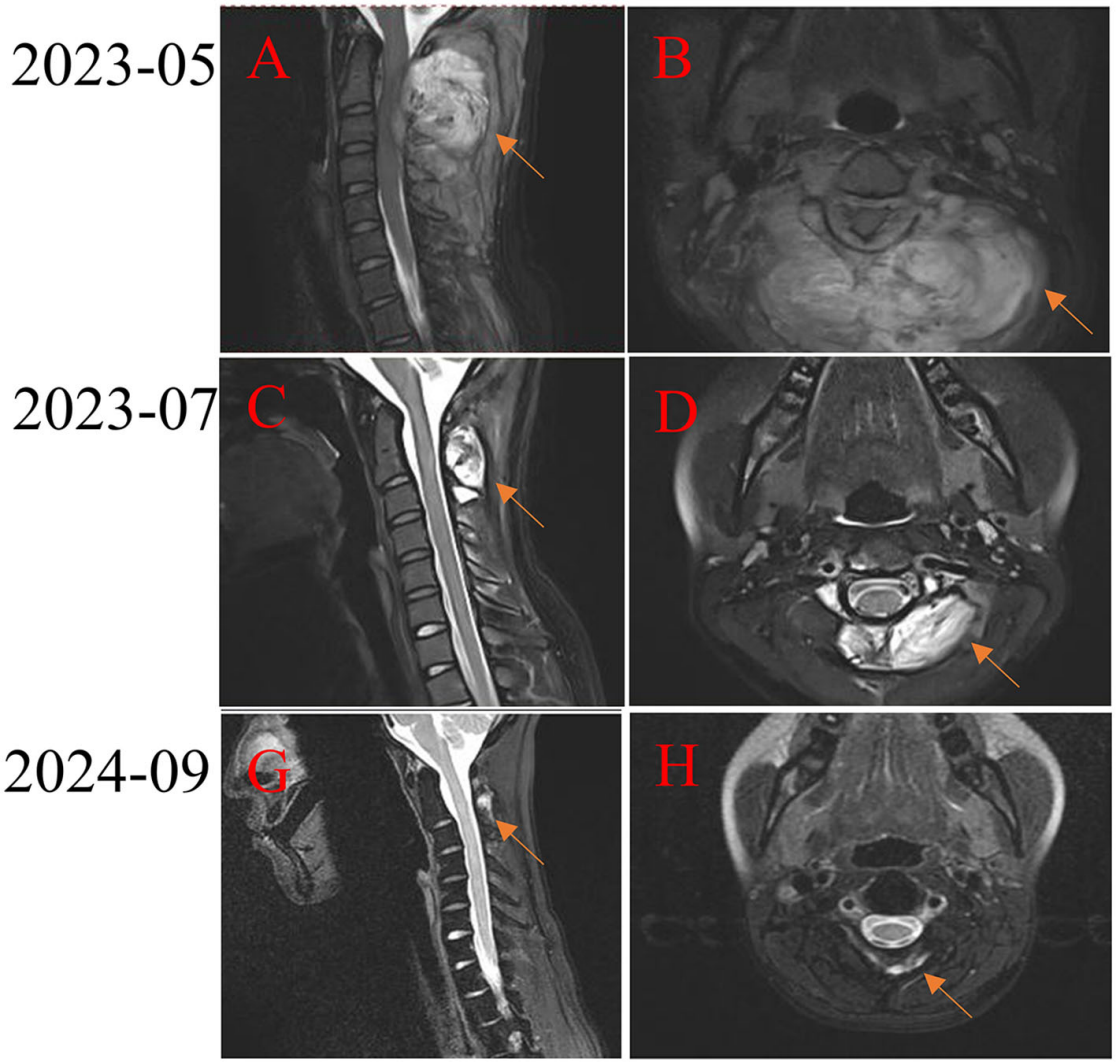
Treatment of a patient with high-grade malignant peripheral nerve sheath tumor of cervical spinal with PD-1 inhibitor. (A, B) Pretreatment MRI scan shows a large tumor in the cervical spinal. (C-H) After receiving PD-1 inhibitor-based combination therapy, the tumor continuously shrinks.
